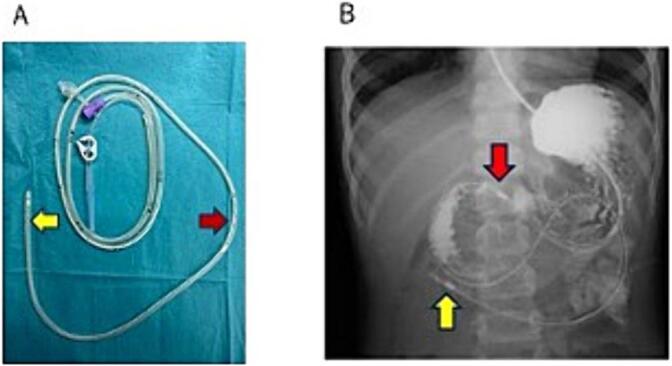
(A)Picture of a double elementary diet tube. The nutrient administration cavity has a hole at the tip(yellow arrow), and the drainage cavity has a drainage hole 40 cm from the tip (red arrow). (B)Abdominal radiographic image after gastrointestinal angiography performed on the 12th day after the injury showed the double elementary diet tube, with the tip placed at the jejunum (yellow arrow) and the drainage marking placed at the stomach (red arrow). (For interpretation of the references to colour in this figure legend, the reader is referred to the web version of this article.)
